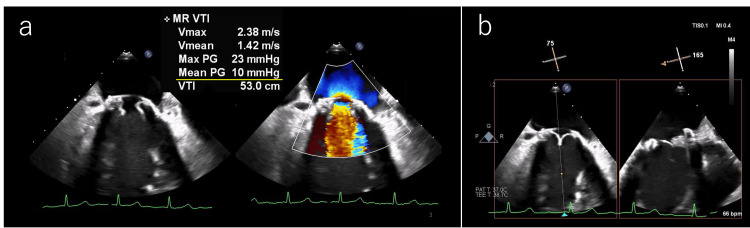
Follow-up transesophageal echocardiography performed seven months after the mitral valve replacement surgery; recurrence of severe mitral stenosis (a), and thrombotic valve formation and immune complex deposition (b)
